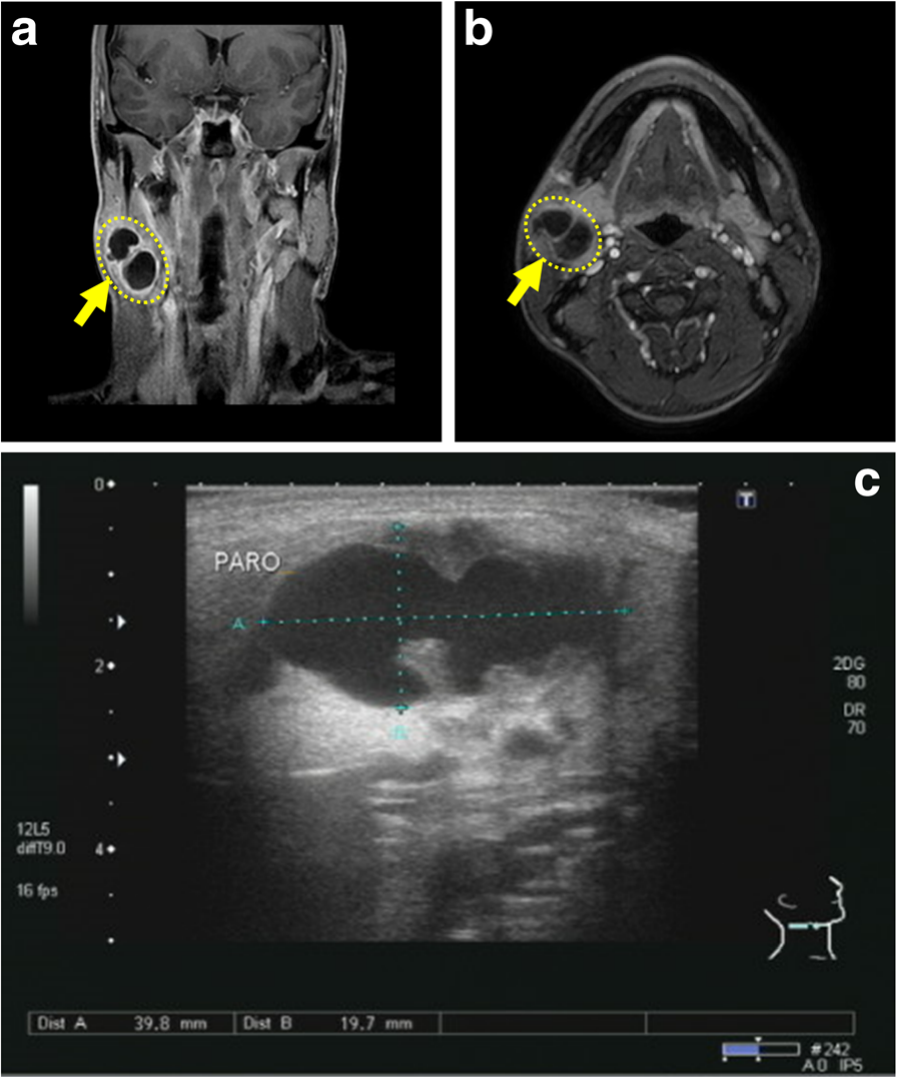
An inflammatory cyst within the submandibular gland. Coronal (a) or axial (b) *T*_2_-weighted MRI images of the head and neck demonstrating the existence of a cyst within the right submandibular gland (yellow arrows). Ultrasonic imaging of the inflamed submandibular gland (c) showed the inflammatory cyst as a black hole with sharp borders, measured 39.8 mm × 19.7 mm in diameter
